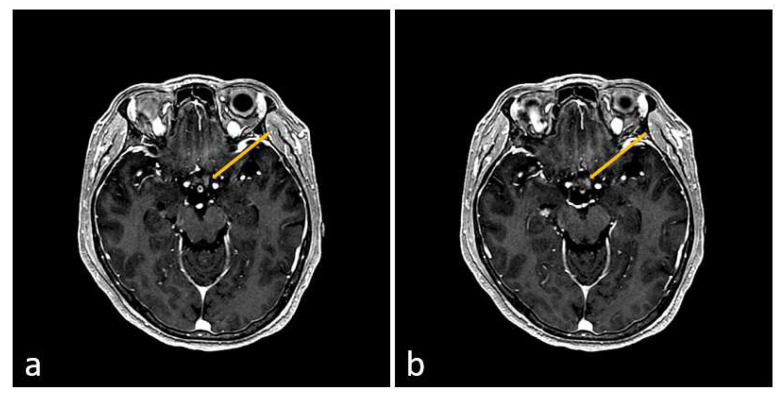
In (a), the arrow indicates high signal intensity of the left optic nerve on the T1 contrast-enhanced image of the brain MRI, and in (b), the arrow indicates high signal intensity of the optic chiasm on the T1 contrast-enhanced image of the brain MRI. These are important imaging findings in the diagnosis of NMOSD.
